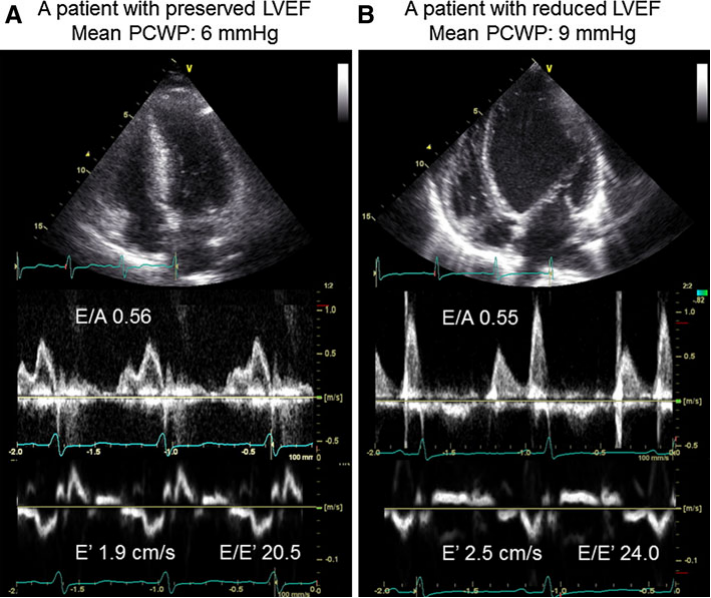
a Apical four-chamber view (*top*), mitral inflow (*middle*), and tissue Doppler-derived mitral annular velocity signals (*bottom*) in a patient with preserved LVEF and normal mean PCWP of 6 mmHg (*left*). b Apical four-chamber view (*top*), mitral inflow (*middle*), and tissue Doppler-derived mitral annular velocity signals (*bottom*) in a patient with reduced LVEF and normal mean PCWP of 9 mmHg (*right*). *LVEF* left ventricular ejection fraction, *PCWP* pulmonary capillary wedge pressure
